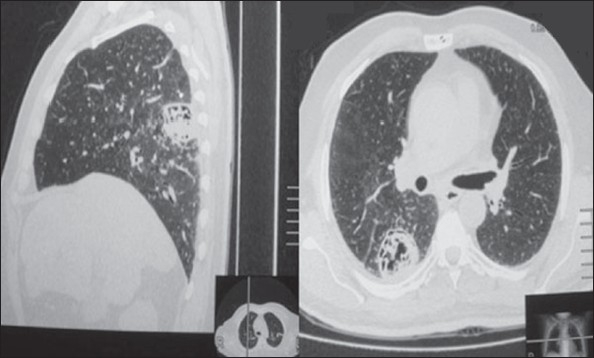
CT scan of the chest showing a cavity in the upper segment of the right lower lobe with lamellar internal echoes.
